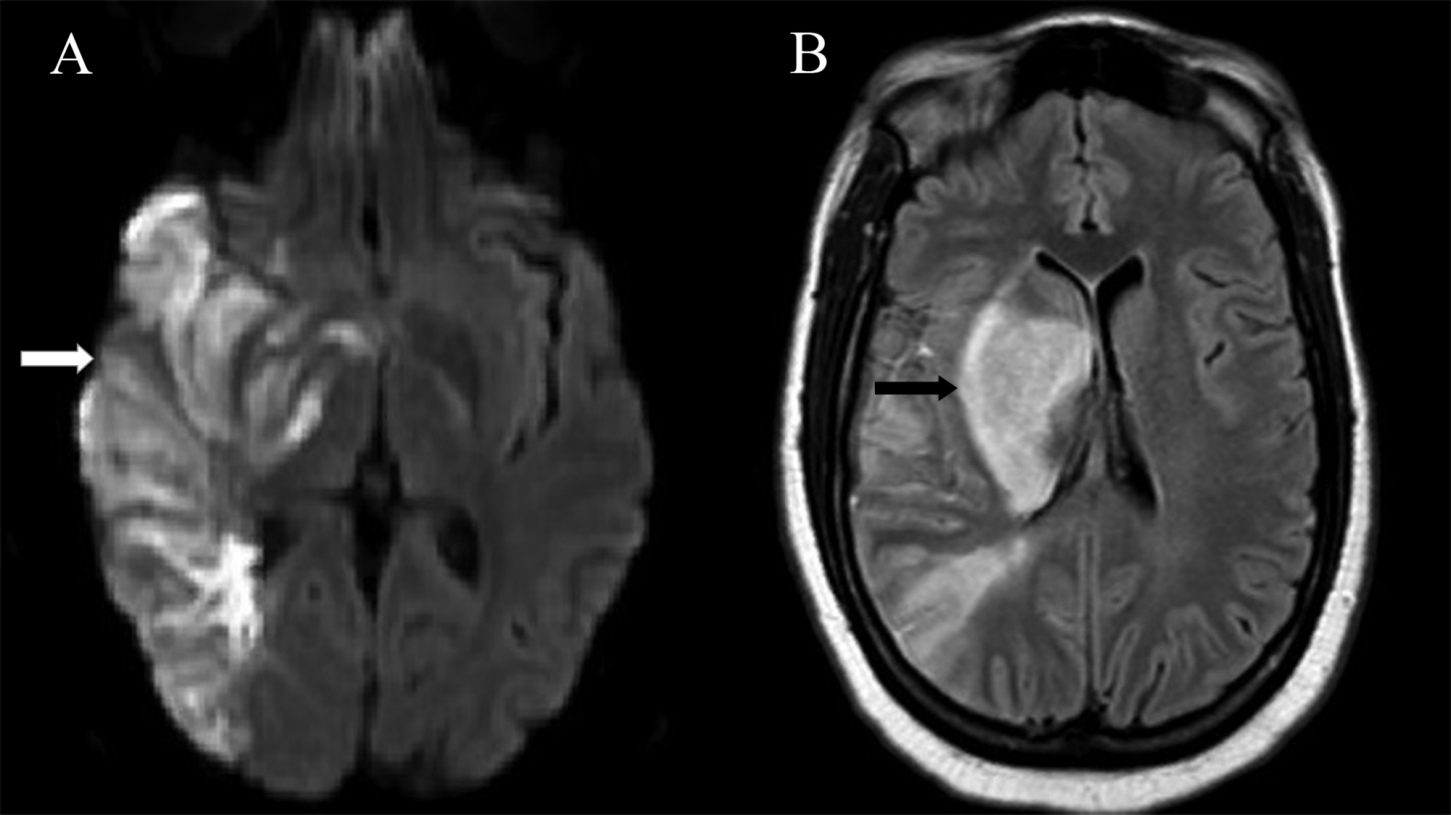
Initial MRI after ischemic stroke A: Axial diffusion weighted imaging (DWI) demonstrating diffusion restriction in the right temporo-parietal lobe (white arrow). B: Axial fluid attenuated inverse recovery (FLAIR) sequence with hyperintensity seen in the area of infarct in the right basal ganglia (black arrow).
